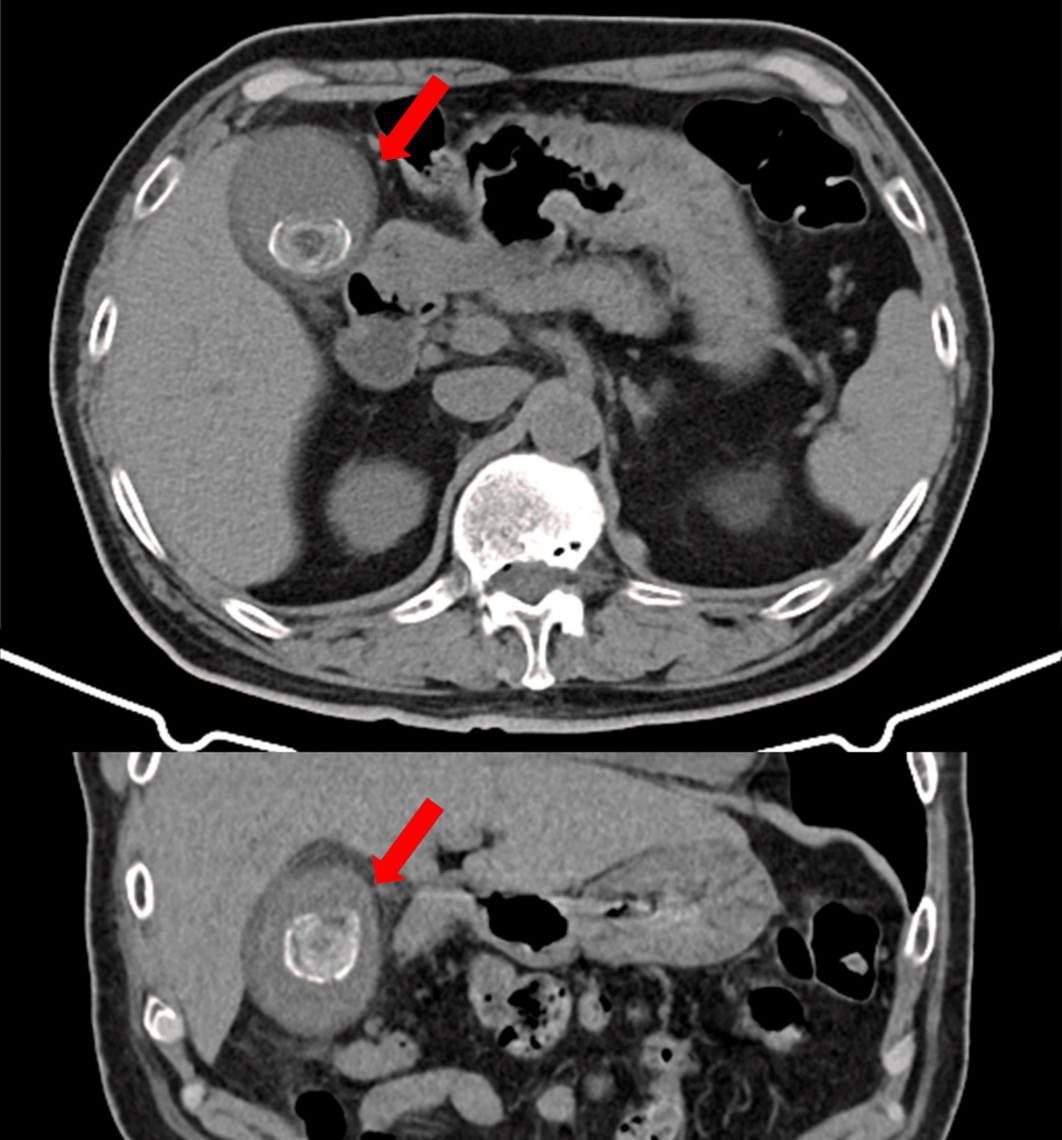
CT findings in Case 3 Chest-abdominal computed tomography showing gallstones with gallbladder enlargement and wall thickening, consistent with acute cholecystitis (red arrow).
